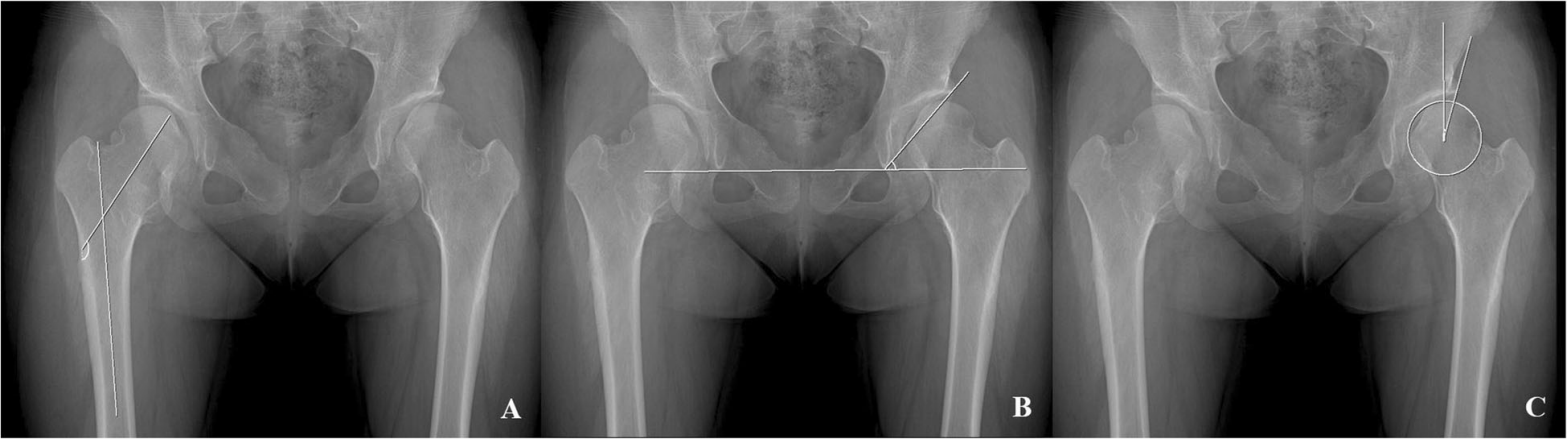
Plain radiographic measurements to evaluate the hip joint deformities. a The femoral neck-shaft angle is determined by measuring the angle created by a line in the central axis of femoral shaft and a second line created by the connection of the femoral head center to the mid portion of the femoral head and neck junction contour. b Sharp’s angle is determined by measuring the angle created by a line connecting the acetabular tear drops and a second line connecting a tear drop and the sourcil end. c The center-edge angle is determined by measuring the angle created by a line connecting the vertical line to the tear drop line through the center of the femoral head and a second line from the center of the hip to the lateral acetabular wall margin
